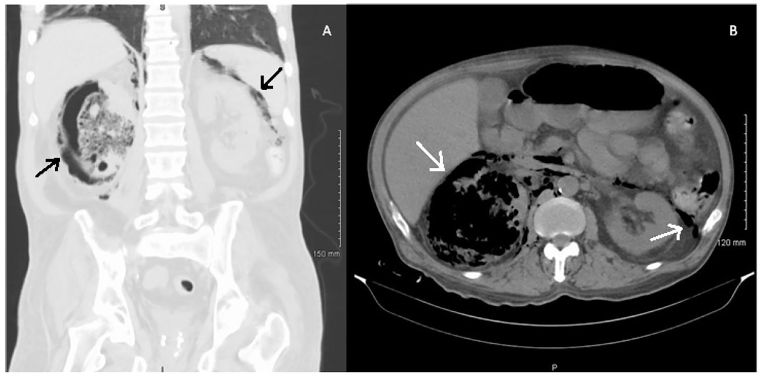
A. Coronal view of a computed tomography (CT) of the abdomen and pelvis, in the lung window, showing bilateral emphysema. B. Axial view of CT of the abdomen and pelvis, without contrast, showing emphysema replacing the right kidney.
